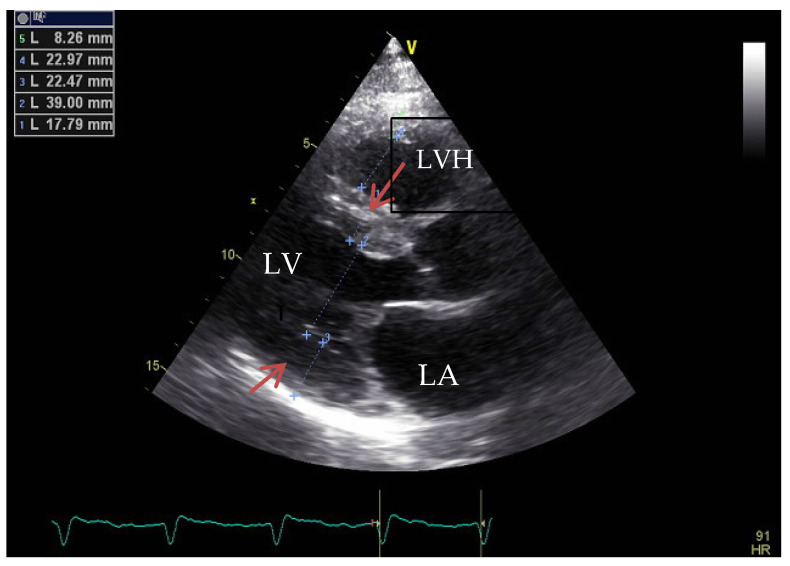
Two-dimensional echocardiography, parasternal long axis view showing an LV with concentric hypertrophy (LVH) and LA enlargement.
